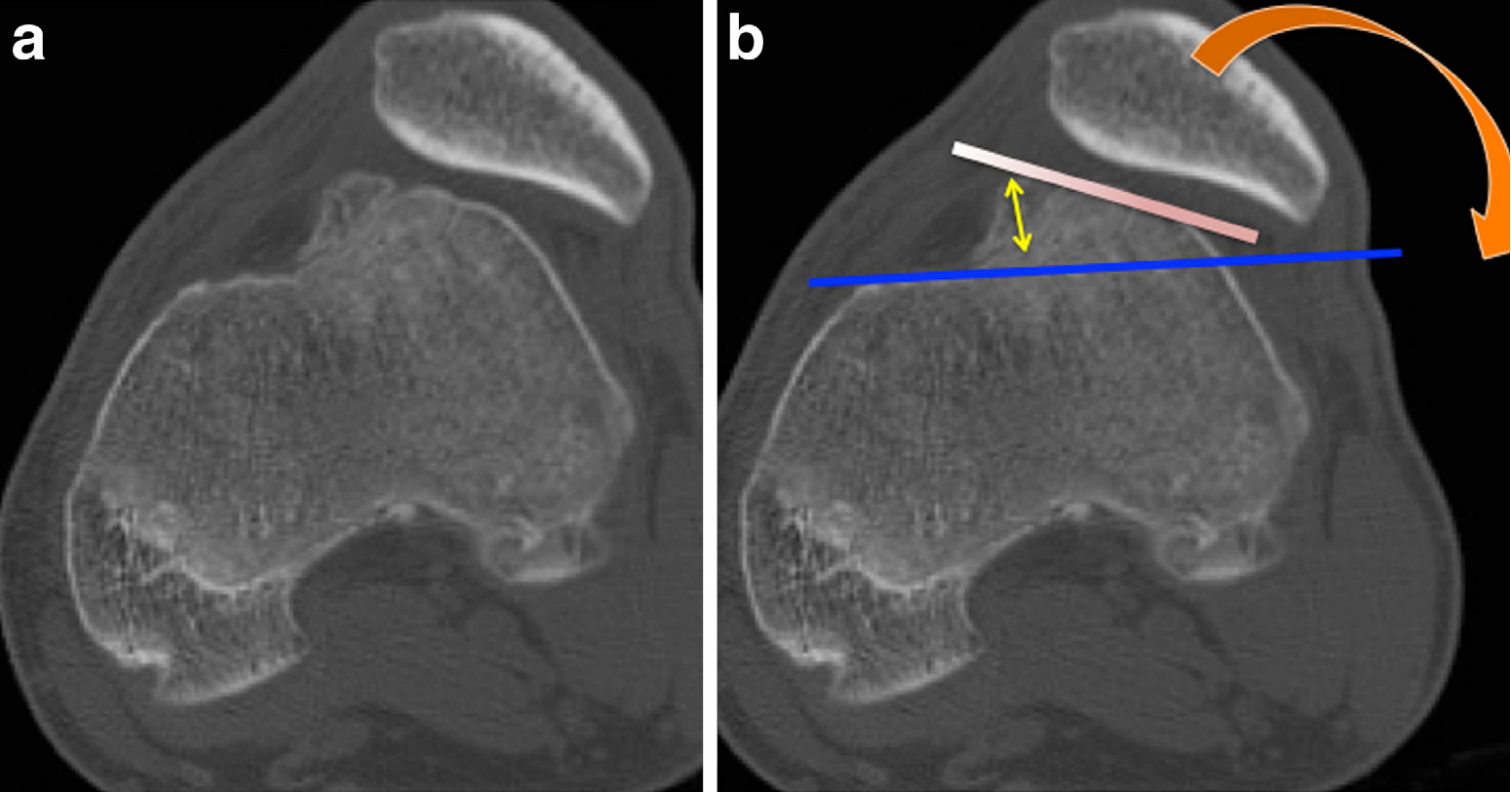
CT scan. It directly evaluates the trochlear prominence. The orientation of the trochlear plane and the trochlear lateralization explain patellar tilt and subluxation.
